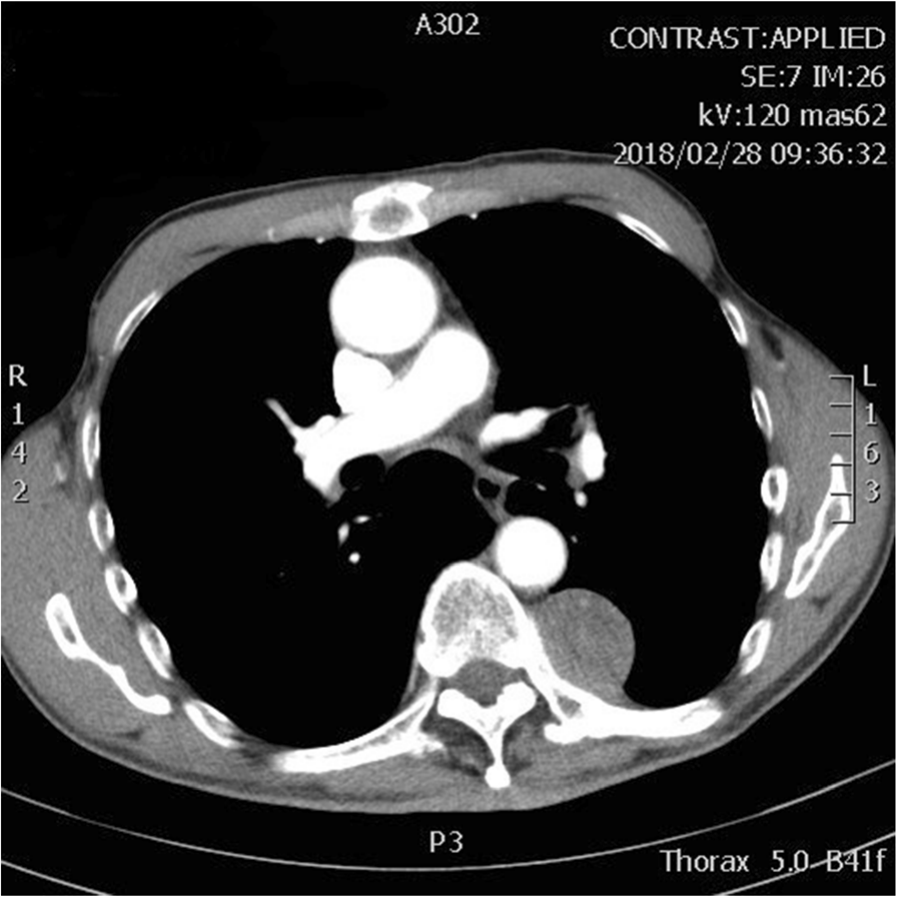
Chest CT. The size of tumor was approximately 4.1 cm × 3.2 cm in the left spinal column, and the enhanced scan showed uniform enhancement
